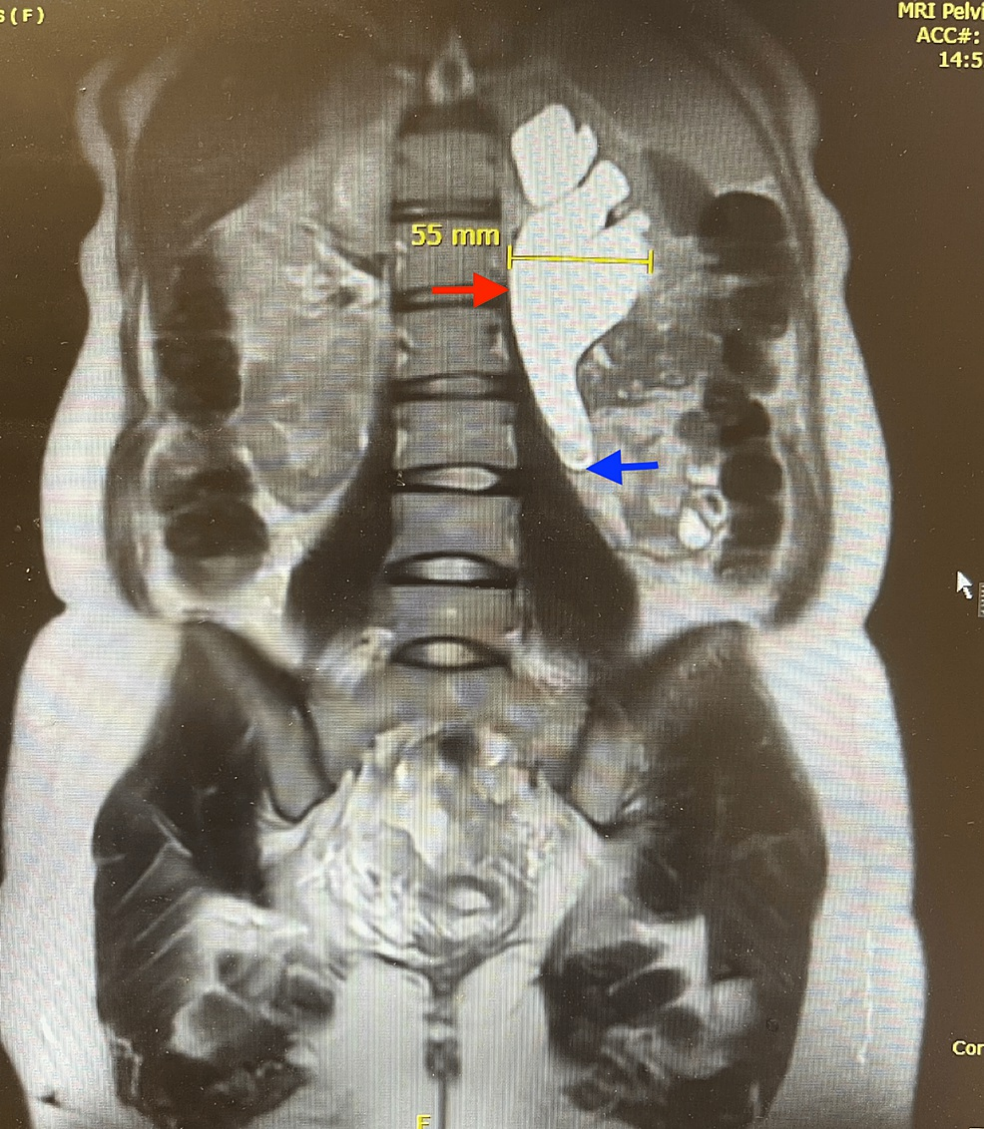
Postoperative MRI shows severe left hydronephrosis. The image shows coronal plane magnetic resonance imaging (MRI) showing severe left hydronephrosis measuring 55 mm (marked with the red arrow) caused by a distal ureteric obstruction from an endometriotic lesion at the point where the ureter abruptly ends (marked with the blue arrow).
